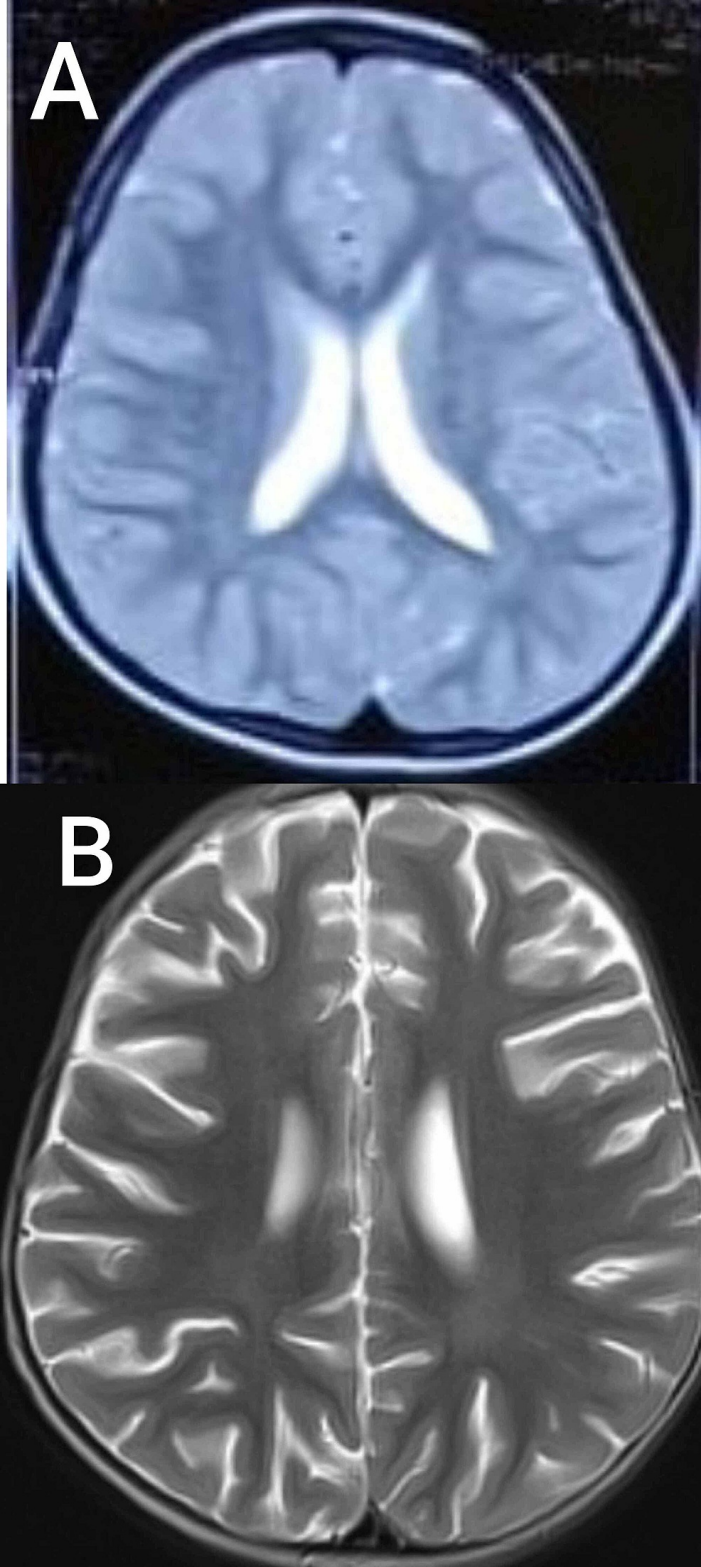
Magnetic resonance imaging (MRI) of the patient's brain (A) Axial T2-weighted image showing normal findings when the patient was one year old. (B) MRI of the brain showing diffuse atrophy when the patient was three years old.
